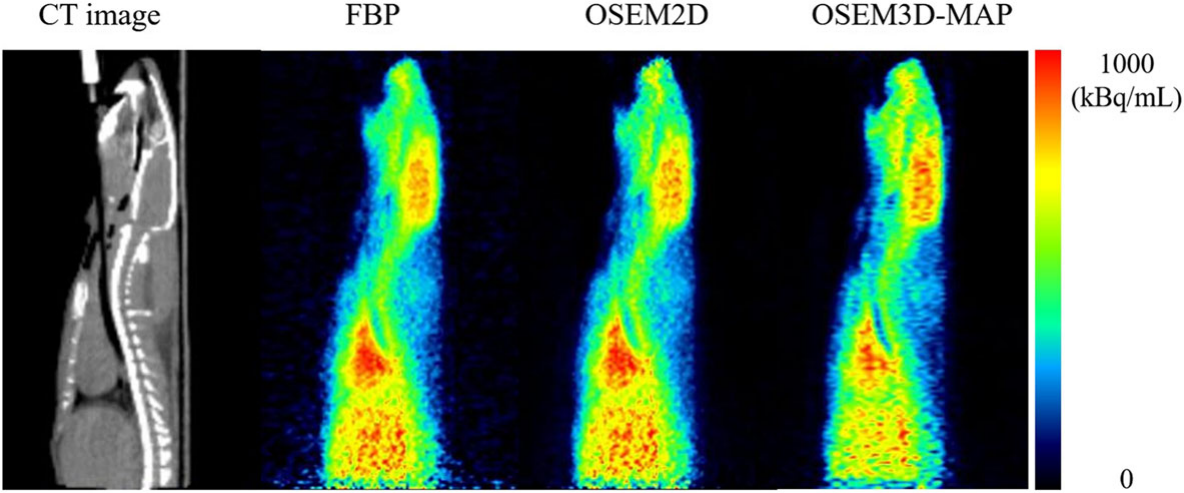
Sagittal PET images reconstructed using FBP, OSEM2D, and OSEM3D-MAP during the steady state in an ^15^O-CO_2_ study and a CT image. The PET image obtained using OSEM3D-MAP showed a higher accumulation in the brain than those images obtained using FBP and OSEM2D based on a visual assessment
